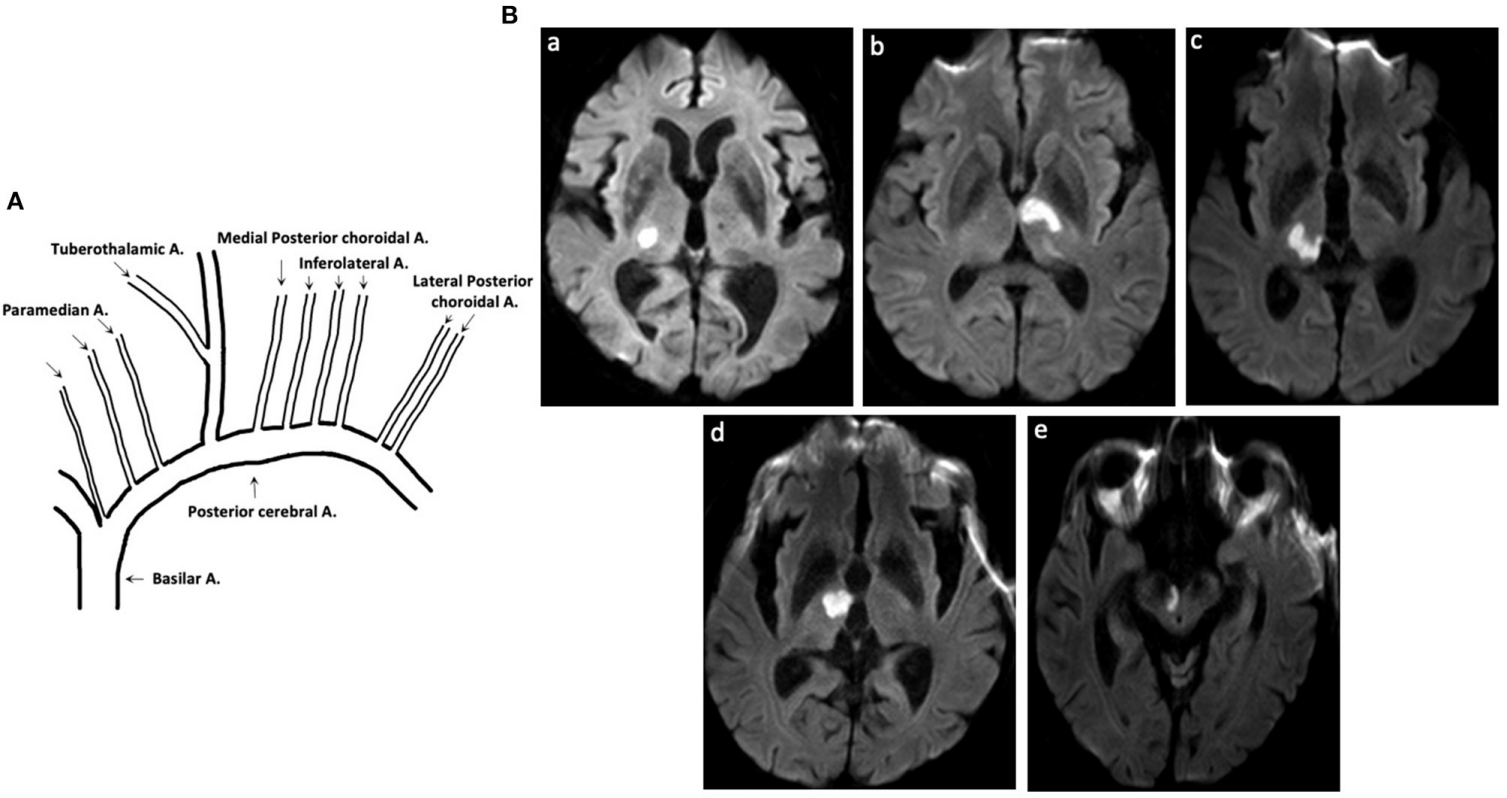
Thalamic blood supplies and DWI of patients with acute pure thalamic infarct. (A) Thalamic blood supplies. (B) DWI-proven acute pure thalamic infarct is shown in the territory of the (a) inferolateral artery, (b) tuberothalamic artery, (c) combination of inferolateral and paramedian arteries, and (d,e) combination of tuberothalamic and paramedian arteries. A, artery; DWI, diffusion-weighted imaging.
